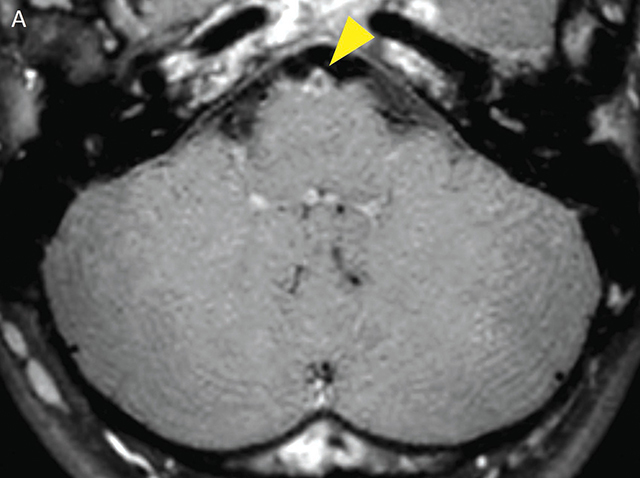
Black-blood-MRI showing eccentric narrowing of the proximal basilar artery and circumferential contrast enhancement in the axial plane (yellow arrowhead).
